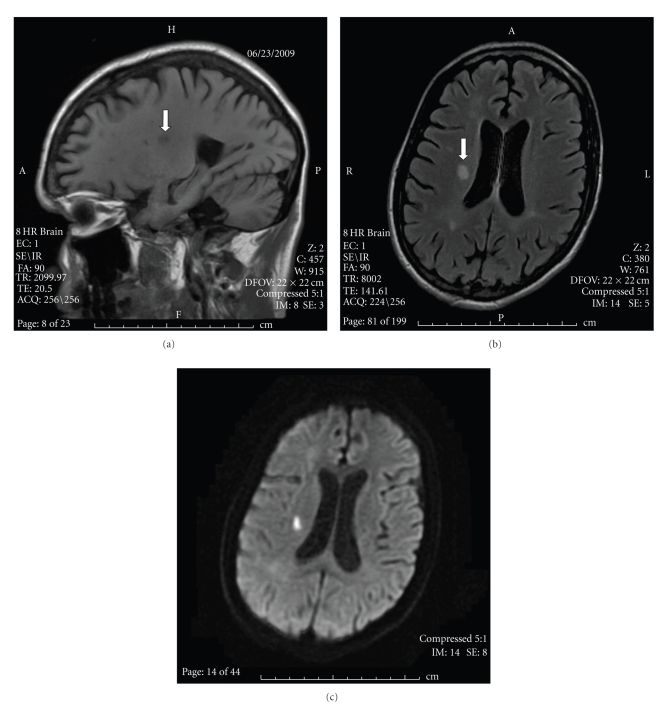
MRI of brain on presentation: (a) sagital view showing acute right side frontal lobe infarct marked by arrow. (b) Axial view with same right side frontal lobe infarct marked by arrow. (c) Diffusion-Weighted Image of ischemic stroke in the right centrum semiovale.
